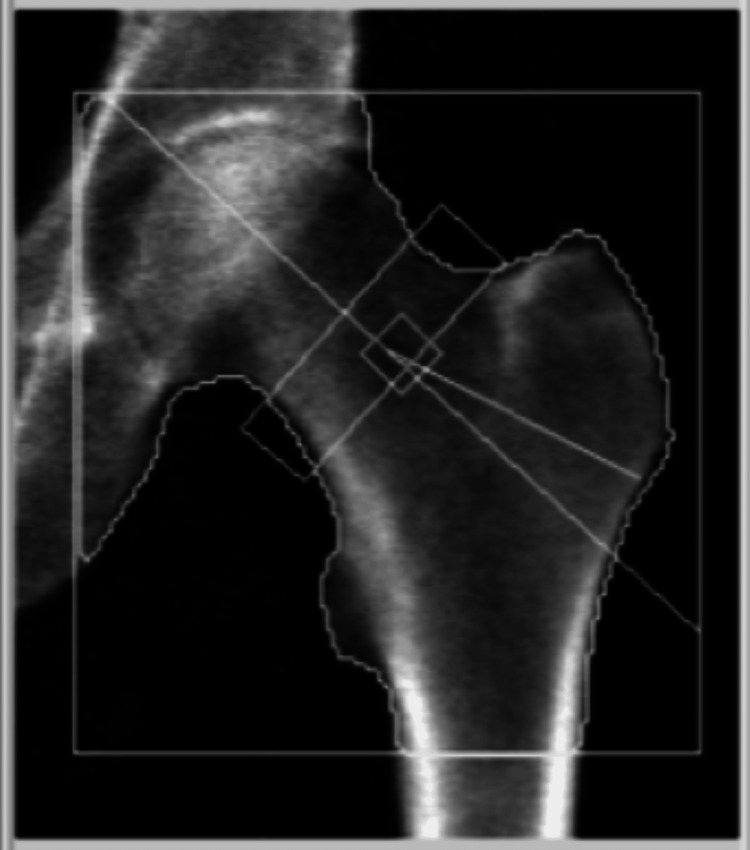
Dual X-ray absorptiometry (DEXA) scan image
